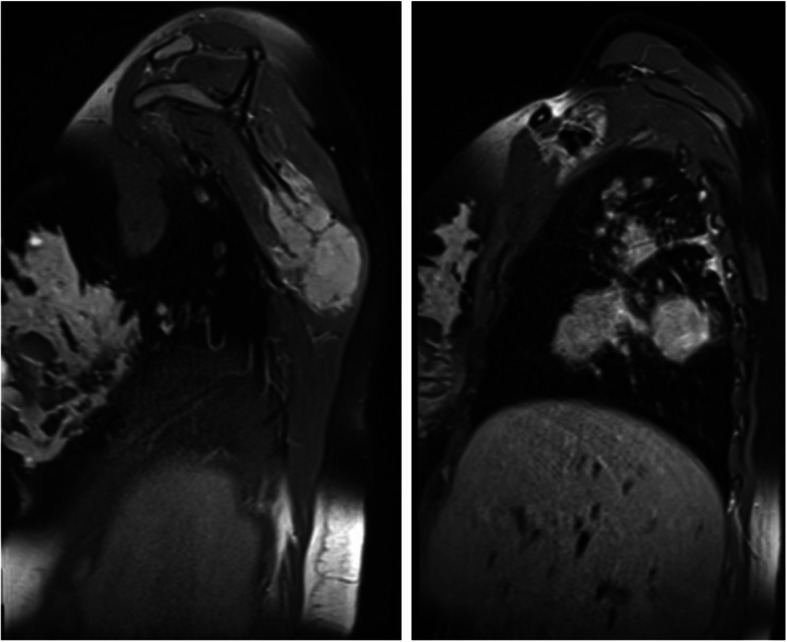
MRI sagittal view showing highly suspicious lesions in the right scapula and lung
